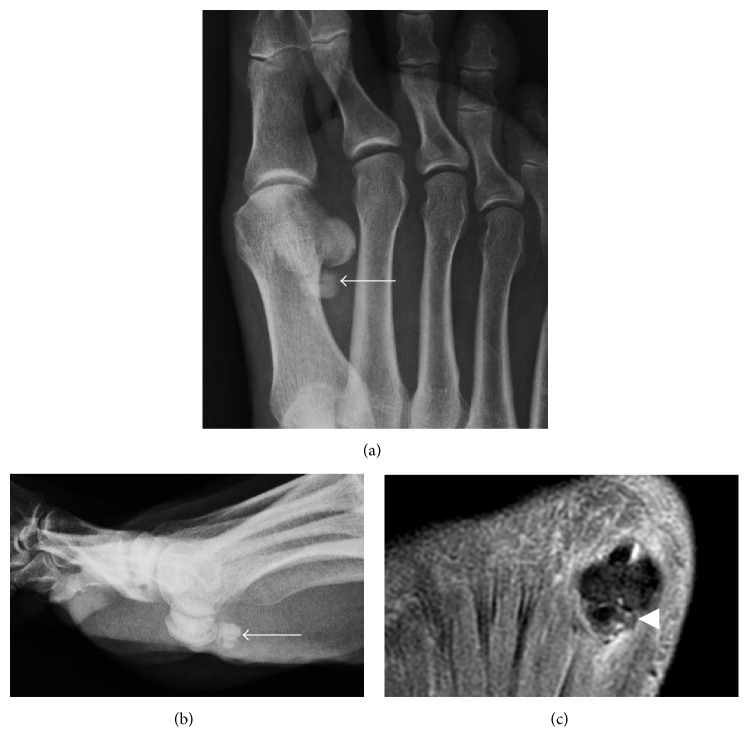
Calcific tendinitis of the flexor hallucis brevis. (a) and (b) Amorphous calcification consistent with acute, symptomatic phase of calcific tendinitis is present just proximal to the hallucal sesamoids (arrows). (c) Long axis PD fat-saturated MR image shows heterogenous signal of the calcification (arrowhead) with mild surrounding edema, which is also consistent with acute symptomatic phase.
